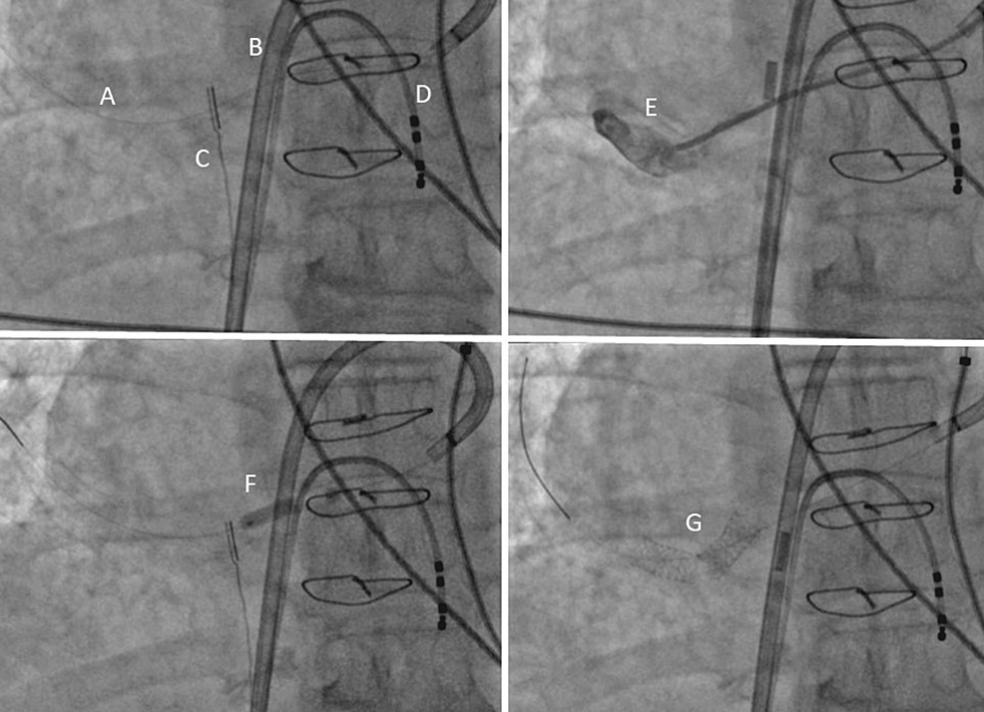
Percutaneous intervention using two stents. (A) Guiding wire in the solitary right pulmonary vein (RPV); (B) steerable sheath trans-septally in the left atrium; (C) intracardiac echocardiography catheter; (D) diagnostic electrophysiology catheter in the right ventricle; (E) angiography of the RPV; (F) inflated balloon in the RPV; (G) two stents in the RPV.
